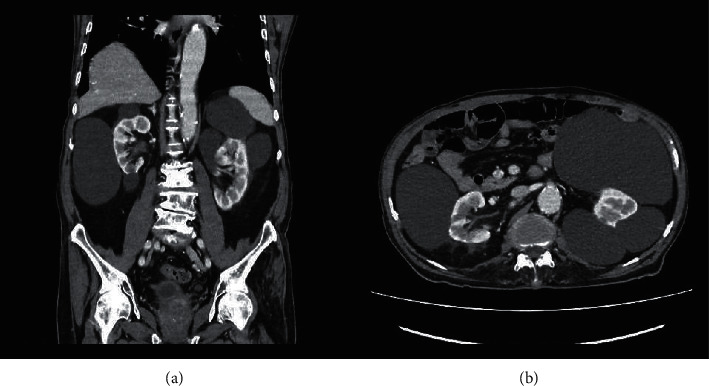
Coronal (a) and axial (b) CT images of the abdomen revealed multiple, variable-sized, thin-walled cysts in both kidneys. The diameter of the cysts ranged from 3.5–13.0 cm. Some renal cysts had higher attenuation with perinephric fat stranding, representing infected renal cysts.
